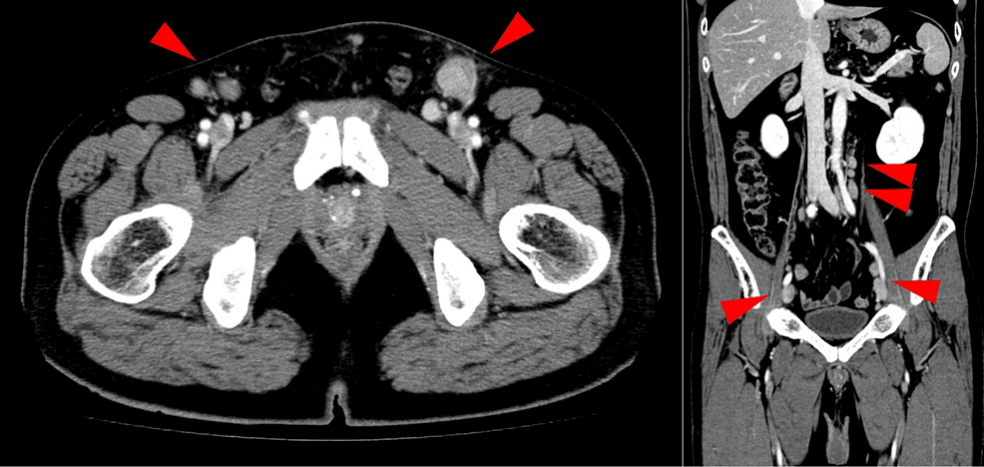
Computed tomography Contrast-enhanced computed tomography showing multiple enlarged lymph nodes in the bilateral inguinal, pelvic, and para-aortic regions taking up contrast (red arrowhead).
